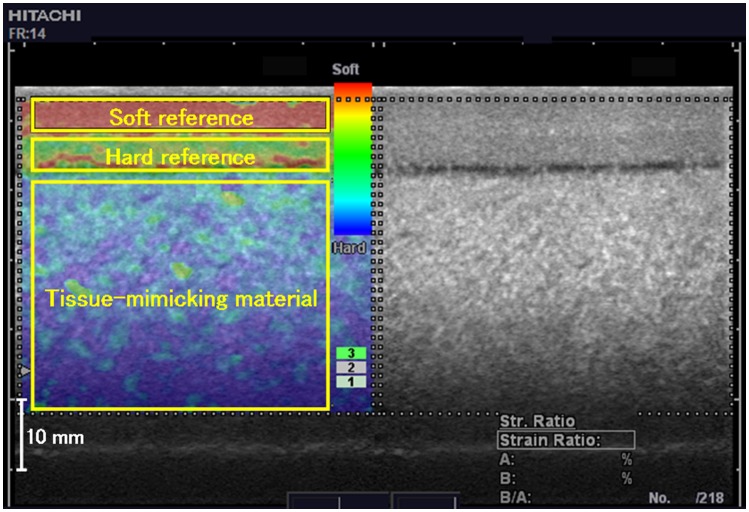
Typical ultrasound elastogram of the tissue-mimicking material. The image on the left is a color-coded elastogram superimposed on a conventional B-mode ultrasound image, whereas that on the right side is the B-mode ultrasound image. On the elastogram, a color-coded scale from red (soft) to blue (hard) and a numeric scale of 1–7 indicating the velocity of the compression–relaxation cycles are presented. Regions of interest within the soft mimicking reference (7 kPa), hard mimicking reference (30 kPa), and tissue-mimicking materials (50 kPa) are indicated by the yellow rectangles.
